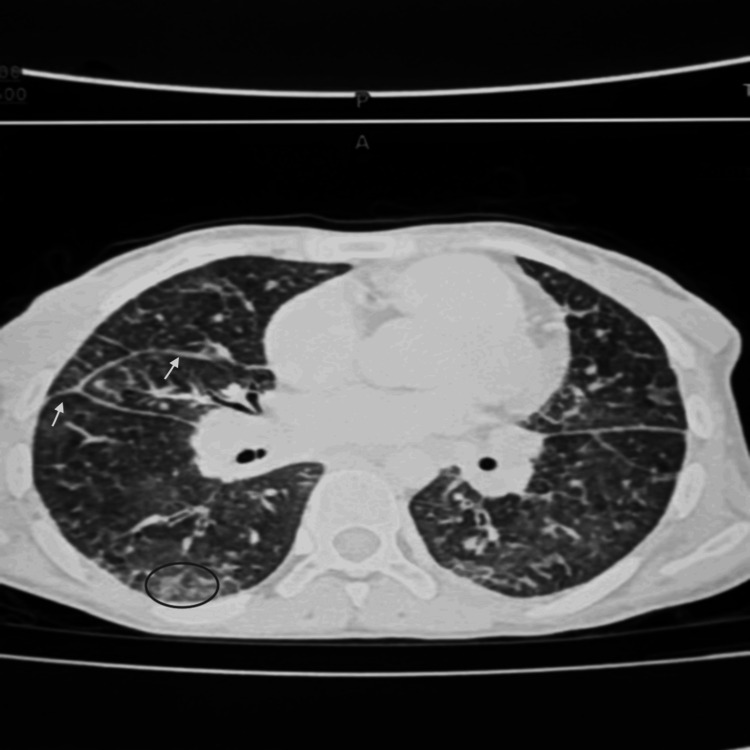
Thoracic CT scan images showing interlobular septal thickening and ground-glass opacities Diffuse bilateral thickening of the interlobular septa, with bilateral areas of ground-glass opacities, more marked in the upper lobes and both basal regions, was observed. Branching centrilobular micronodules and parenchymal nodules were present. Mediastinal lymphadenopathy involving the subcarinal region, para-aortic area, Barety’s space, and bilateral hilar regions was present. Bilateral pleural effusion and right fissural pleural effusion, associated with a mild to moderate pericardial effusion, were observed.
